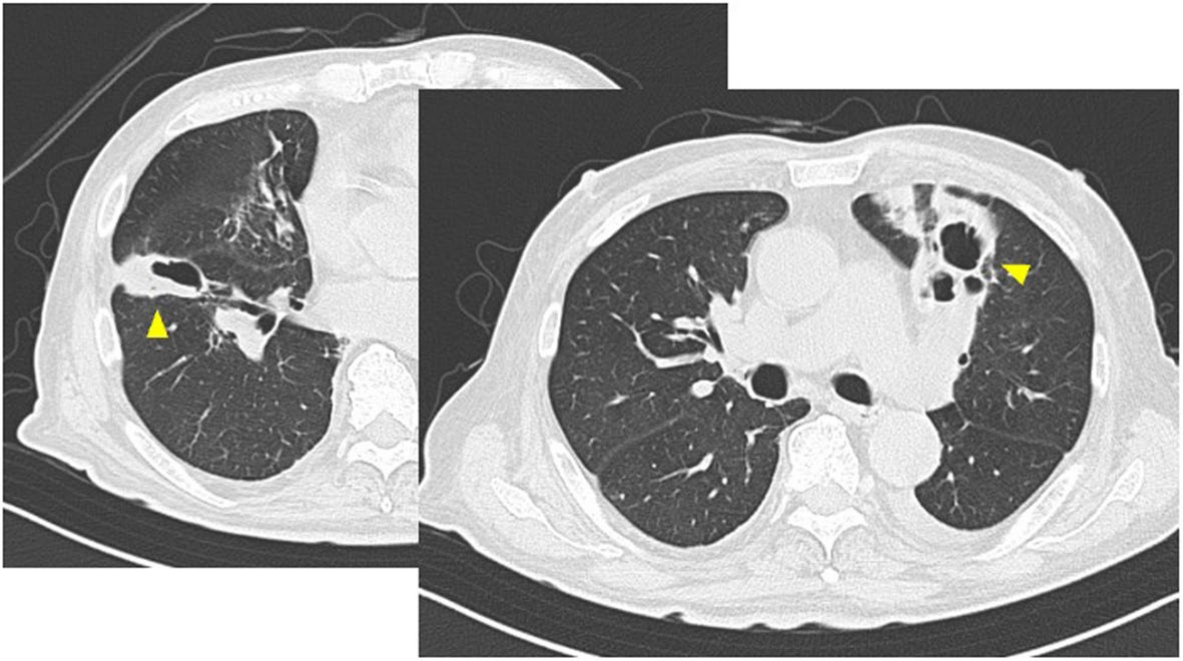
Preoperative chest CT shows multiple bilateral lung abscesses (arrowheads)
